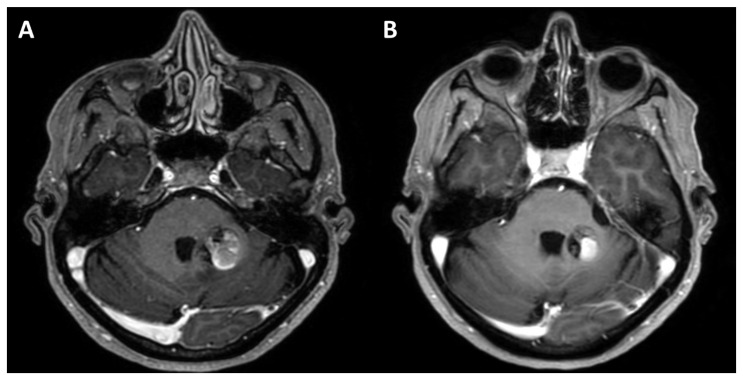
Axial MR images of acute CCM hemorrhage (A) after chronification (B) before surgery.
